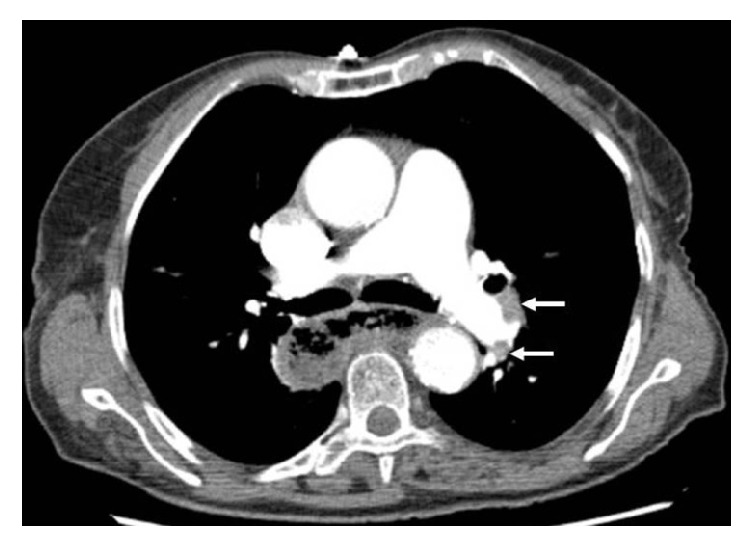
Computed tomography angiography showing proximal pulmonary emboli (white arrows).
